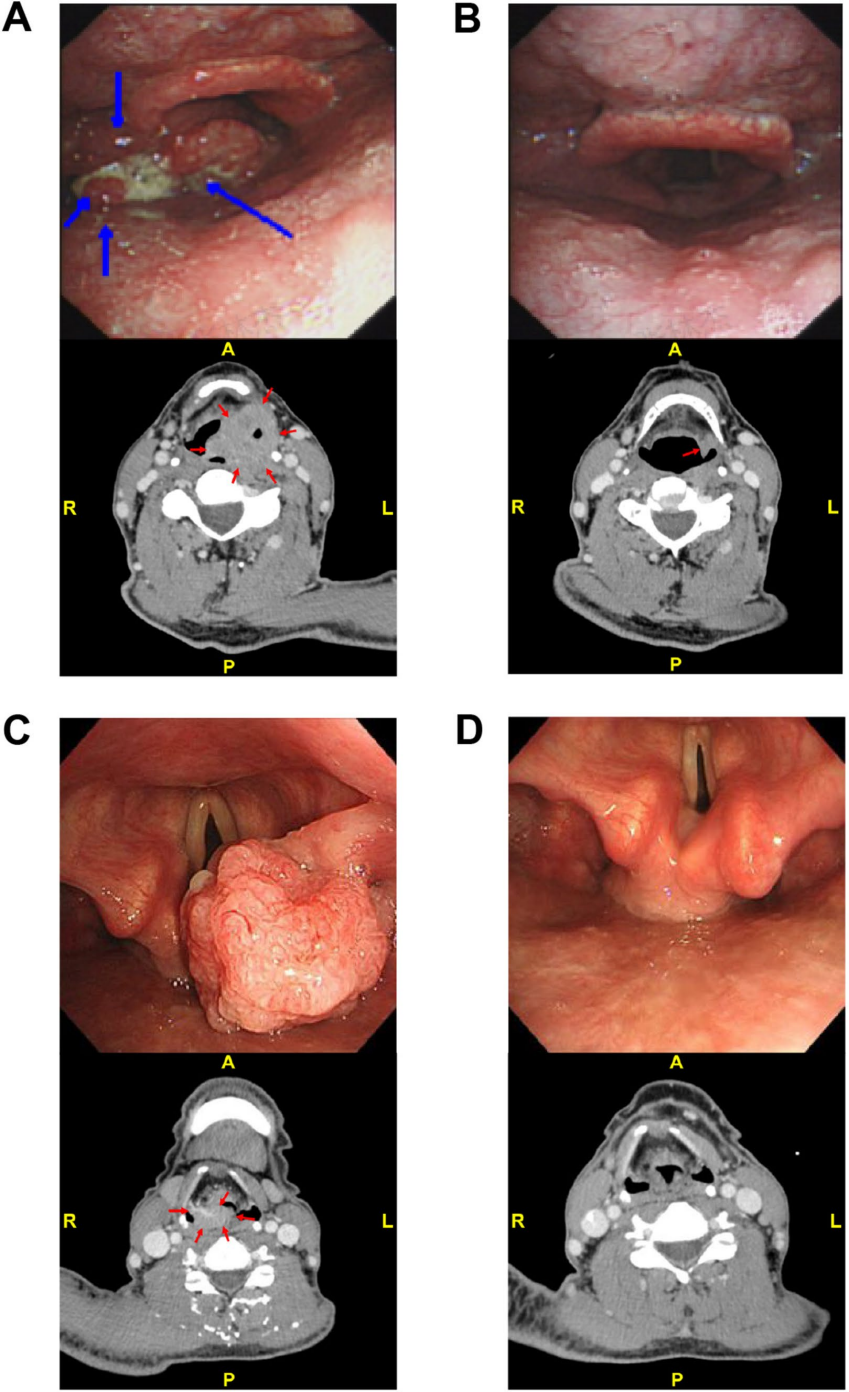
Exemplary Cases of Tumor Regression. A Hypopharyngeal SCC (Patient 36, age 62, T4N2bM0, Stage IV); endoscopy and CT identified a left pyriform sinus cT4 SCC. B After three cycles of neoadjuvant Camrelizumab + TP therapy, imaging indicated a partial response (PR) of the primary lesion. C Supraglottic laryngeal SCC (Patient 56, age 55, T3N2bM0, Stage IV); endoscopy and CT identified a right aryepiglottic fold cT3 SCC. D After three cycles of neoadjuvant Camrelizumab + TP therapy, imaging indicated a complete response (CR) of the primary lesion. SCC, squamous cell carcinoma
